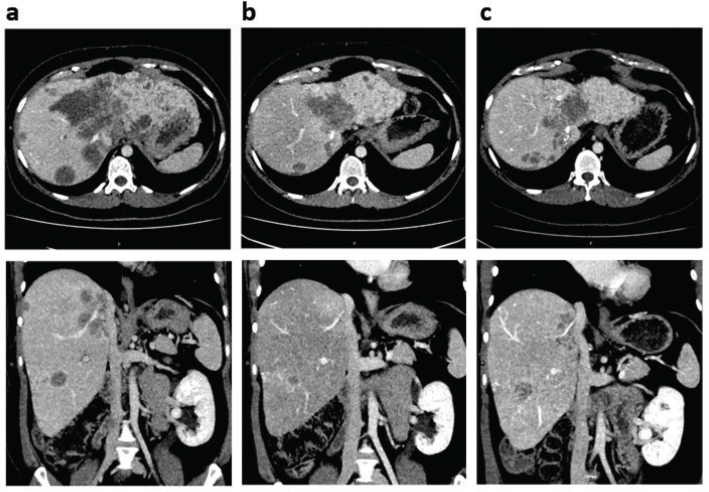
Partial response followed by disease progression on pemigatinib in the patient with aggressive, chemotherapy-resistant CCA. CT images show (a) disease progression after three cycles of GemCis chemotherapy, (b) partial response after three cycles of pemigatinib, and (c) disease progression after seven total cycles of pemigatinib. Axial and coronal views are shown on the top and bottom rows, respectively. CCA: cholangiocarcinoma; CT: computed tomography; GemCis: gemcitabine + cisplatin.
